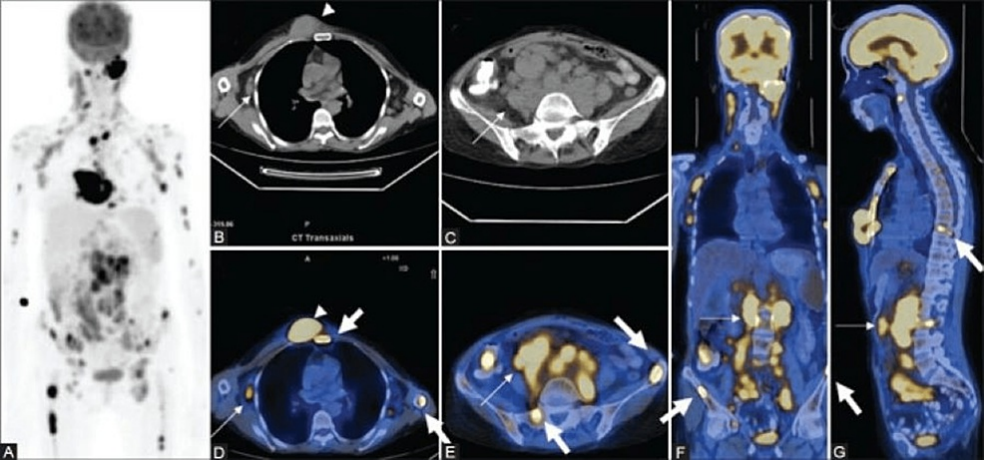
Use of PET/CT in the staging of lymphomas including the ability to diagnose bone marrow involvement Maximal intensity projection image (A) in a patient of diffuse large B-cell lymphoma shows extensive sites of involvement visualized as areas of increased fluorodeoxyglucose uptake. Trans-axial computed tomography images (BB, C show axillary and abdominal lymphadenopathy (thin arrows) ad a large subcutaneous nodule (arrowhead). Fused PET/CT images (axial D, E; coronal F; and sagittal G) show sites of bone marrow involvement (thick arrows) in the sternum, spine, and iliac crest, over and above the lesions picked up on CT This image is reproduced from D'souza et al. [64] - available via Creative Commons Attribution - Noncommercial- Share Alike 3.0 Unported license
